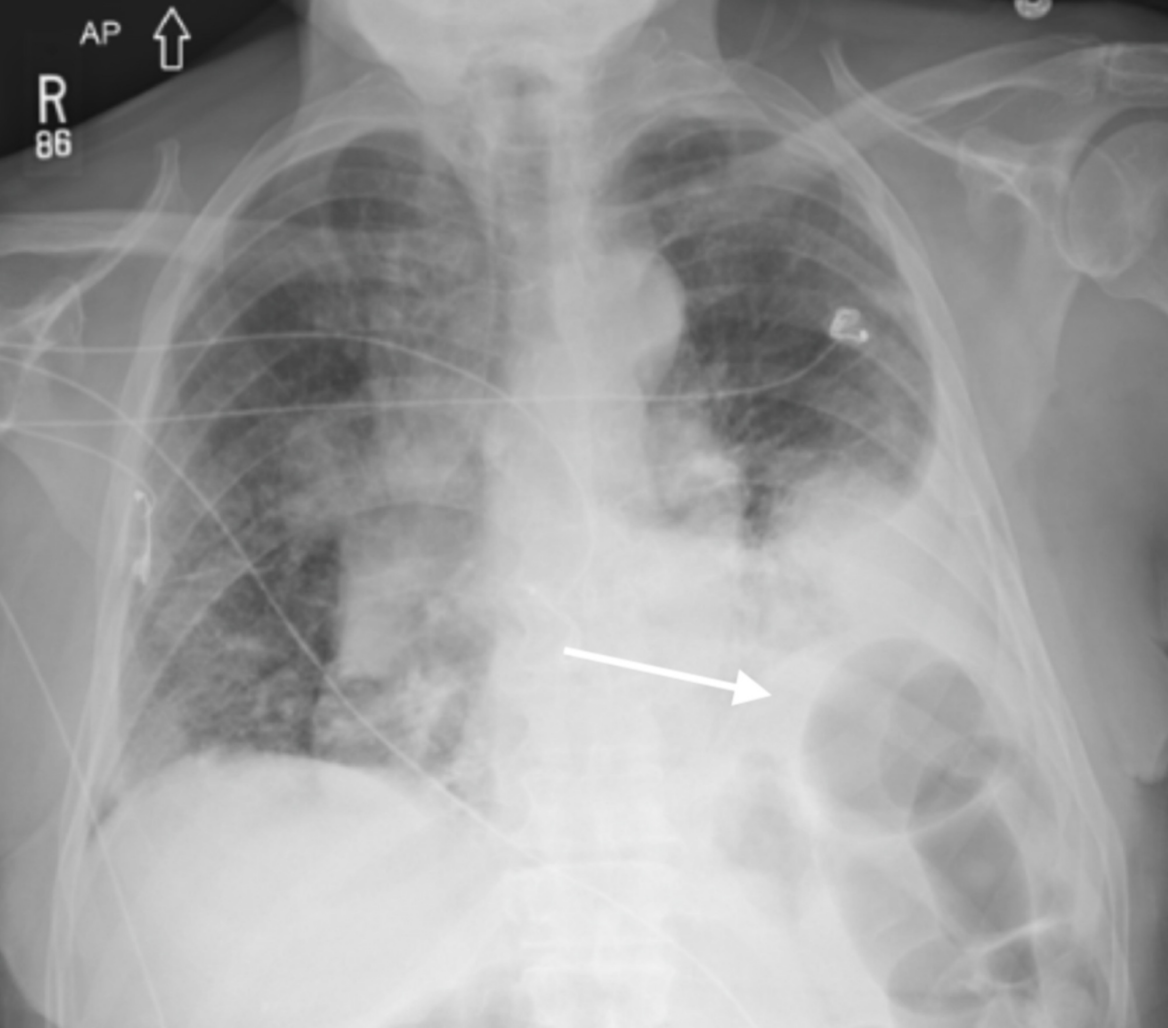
Upright chest X-ray revealing a dense airspace opacity at the left lung base (white arrow) suggestive of pleural effusion.
